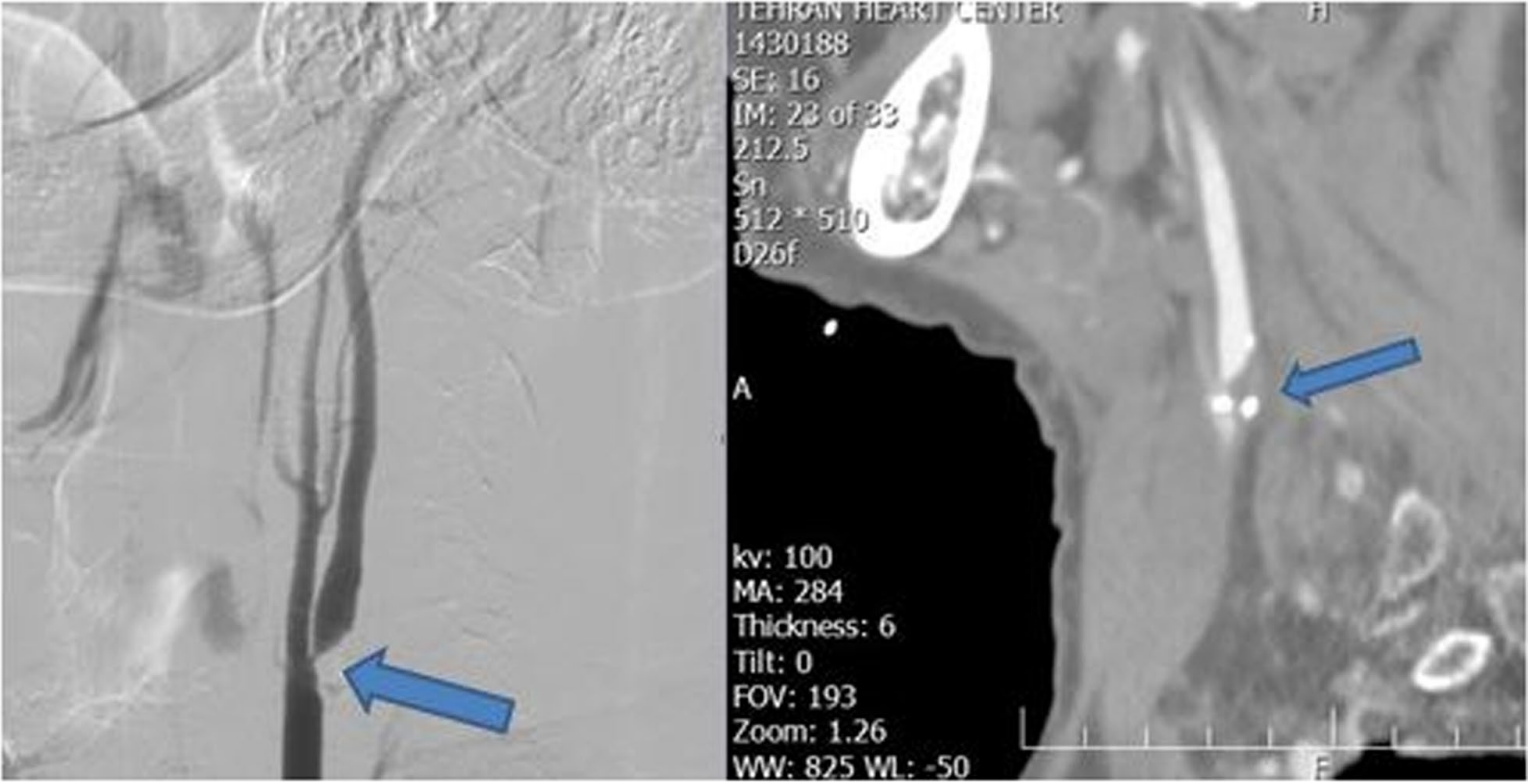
Before stenting: Left image, severe stenosis in origin of left internal carotid artery. Right image, CT angiography and calcification of LICA
